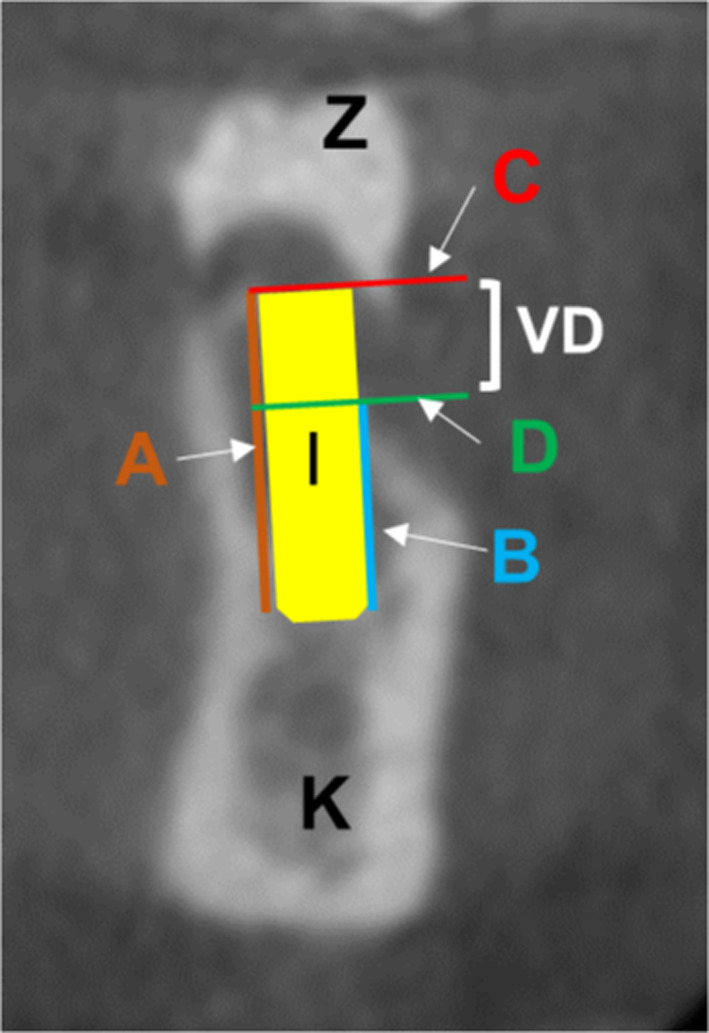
CBCT coronally slice: A and B lingual and buccal lines parallel to the implant axis, C and D lines perpendicular to A and B in order to determine the vertical distance (VD), K jawbone, I of the virtually planned implant
